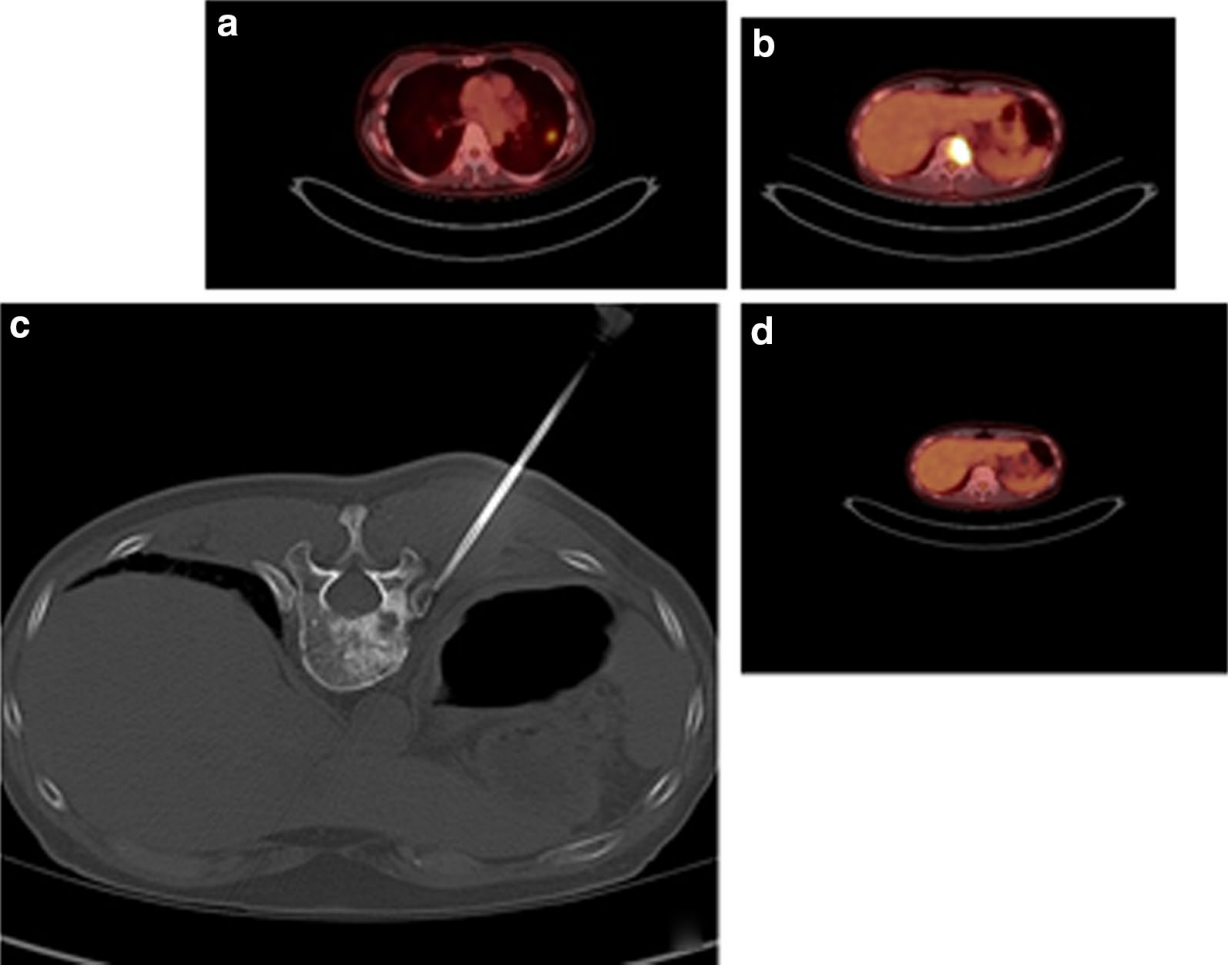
a Diagnostic imaging from September 2013, 2 cm left lower lobe lesion, multilevel mediastinal involvement, and numerous hypermetabolic skeletal lesions. b May 2014 PET-CT demonstrating new hypermetabolic lesion at T12. c January 2015 attempted CT guided biopsy of T12 lesion. d August 2015 PET-CT demonstrating response to third generation TKI following identification of *EGFR* resistance mutation
